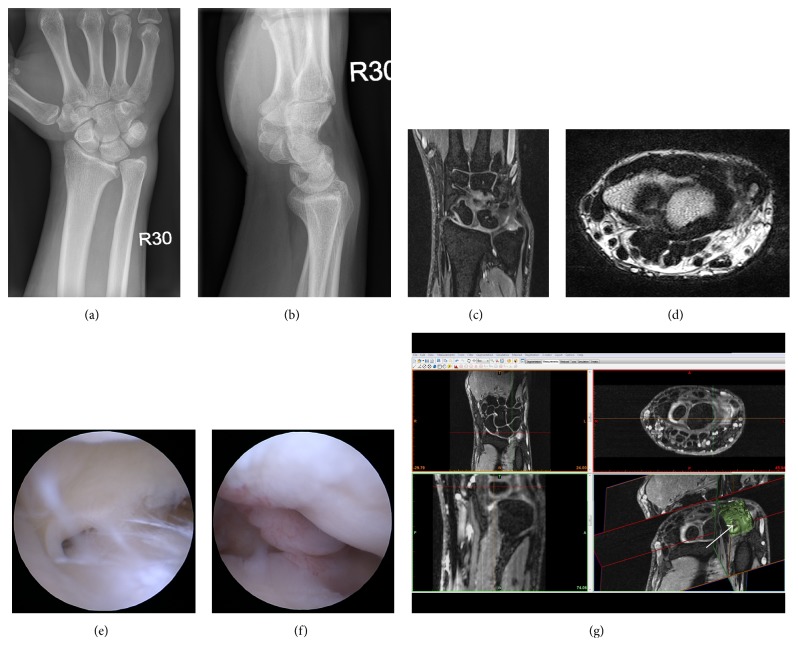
Case of a 31-year-old male. (a, b) X-ray of the right wrist: anterior-posterior (a) and lateral (b) view. (c, d) T2-weighted magnetic resonance imaging in the sagittal (c) and coronal (d) plane, suspected diagnosis Palmer 1B lesion. (e, f) Hand arthroscopy of the same patient, showing a Palmer 2C lesion (e) and injected synovial villi at the radioscapholunate ligament (f). Figure 5(g) shows all anatomical planes in 1.5 T magnetic resonance imaging (top row, bottom left) and a three-dimensional reconstruction of the ulnocarpal disc region (bottom right) of the same patient. The defect cannot be visualized clearly in the standard planes from the MRI. The arrow points at the lesion in the ulnocarpal disc in the segmentation-based reconstruction, indicated by the flattened groove surrounded by two bulky tissue remainders on either side.
